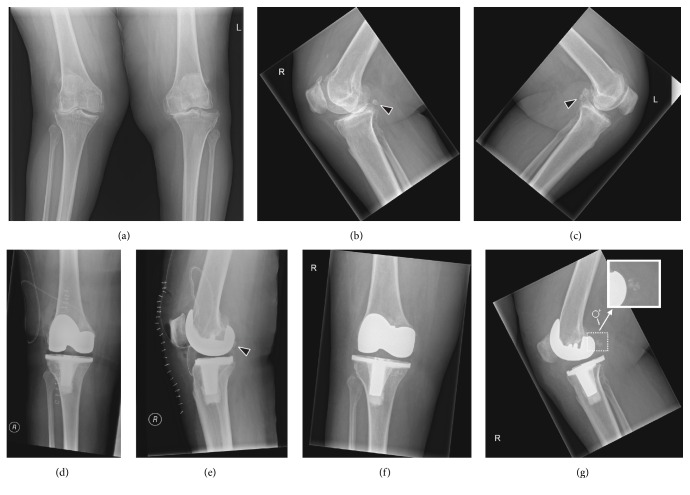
Preoperative (a–c), immediate postoperative (d, e), and 45-day postoperative (f, g) knee radiographs in a 63-year-old woman who underwent total knee arthroplasty of the right knee because of symptomatic knee osteoarthritis with valgus malalignment. Preoperative knee radiographs (anterior-posterior (a) and lateral views (b, c) of both knees) show osteophytes, sclerotic changes, and severe joint space narrowing of right-sided lateral and left-sided medial femorotibial compartments, in keeping with femorotibial osteoarthritis. There is approximately 23° valgus malalignment of the right knee and approximately 7° varus malalignment of the left knee. Note the normal appearing fabellae ((b) and (c) arrowheads). Immediate postoperative radiographs of the right knee on the same day of surgery (anterior-posterior view (d) and lateral view (e)) show a good position of the total knee arthroplasty with a well-aligned knee joint and with a still normal appearing fabella ((e) arrowhead). However, 45-day postoperative radiographs of the right knee (anterior-posterior view (f) and lateral view (g)) show a shattered patella ((g) including magnified view of the fabella).
